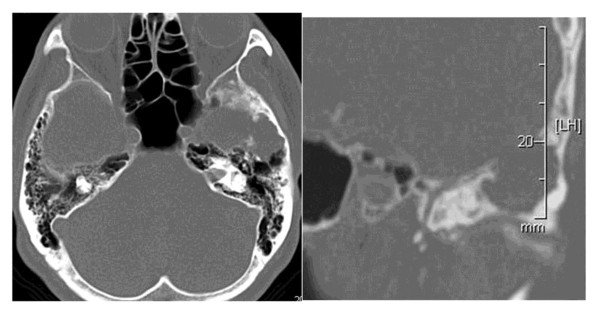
Bone computed tomography images demonstrate a bone-eroded lesion at the middle cranial fossa.
